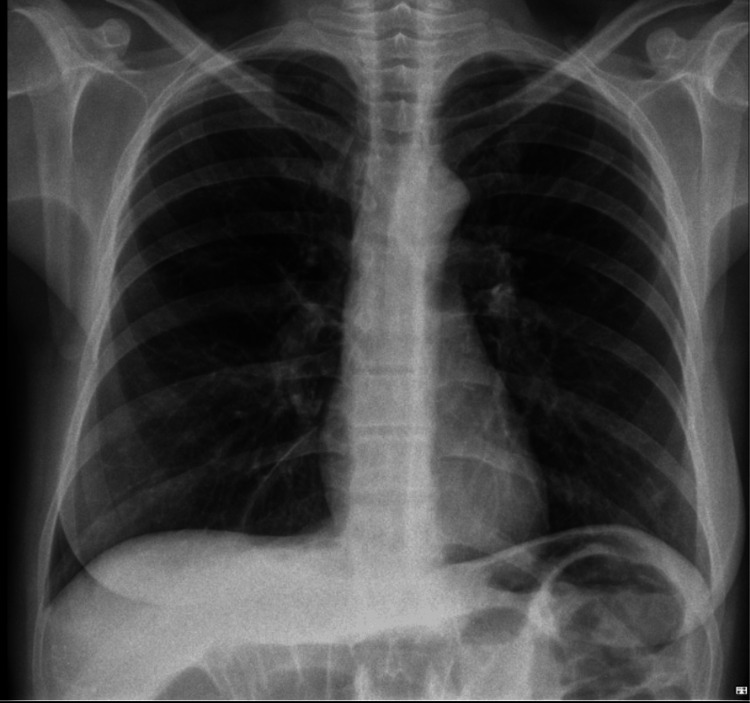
Posteroanterior chest X-ray without alterations
